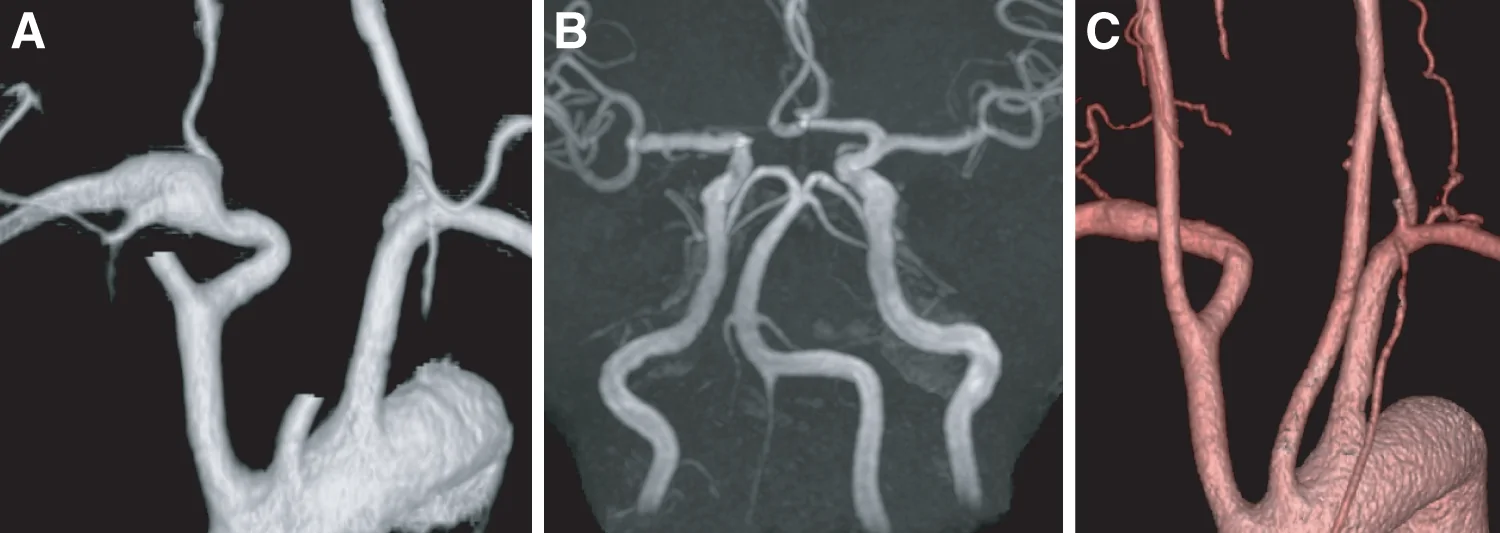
(A) Preoperative MRA reveals a right subclavian artery aneurysm at the vertebral artery origin. (B) Preoperative MRA reveals a dominant left vertebral artery. (C) Postoperative CTA shows good patency of the reconstruction. MRA, magnetic resonance angiography; CTA, computed tomography angiography
